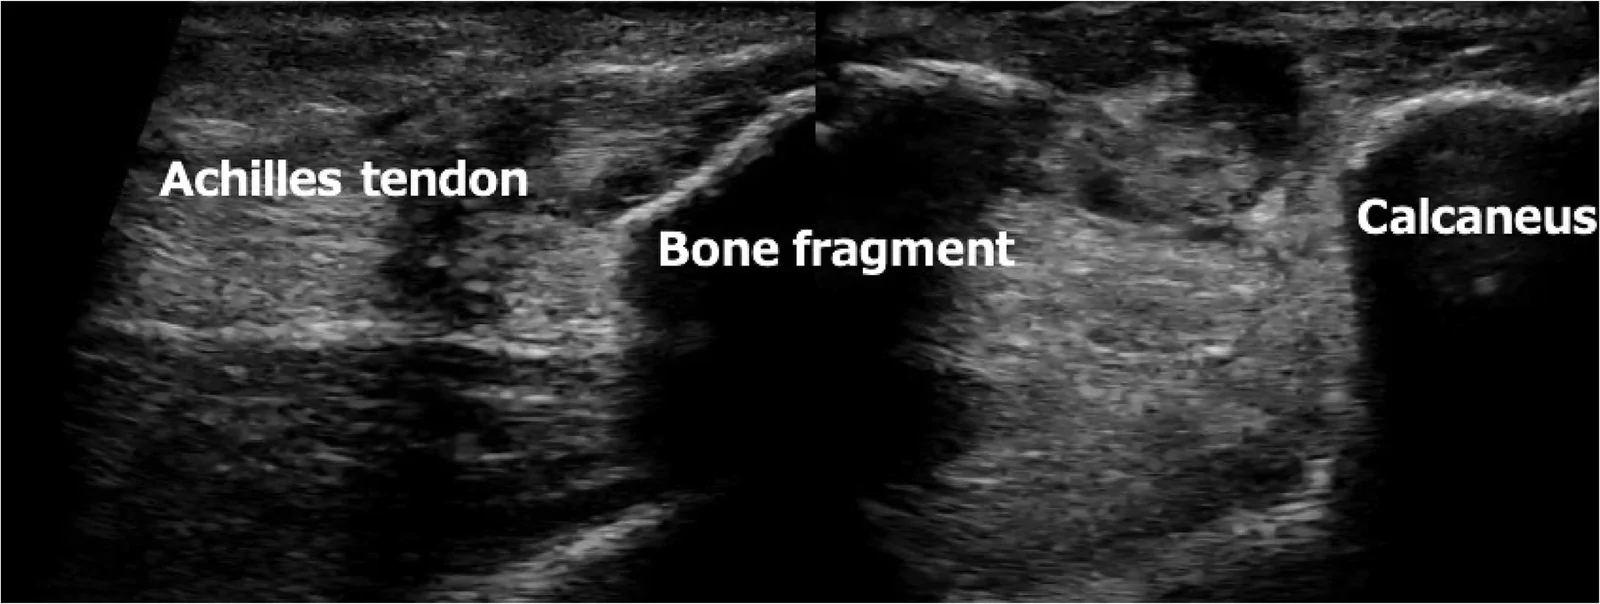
Preoperative ultrasonography demonstrating the Achilles tendon attached to the avulsed bone fragment with discontinuity from the calcaneal body.
